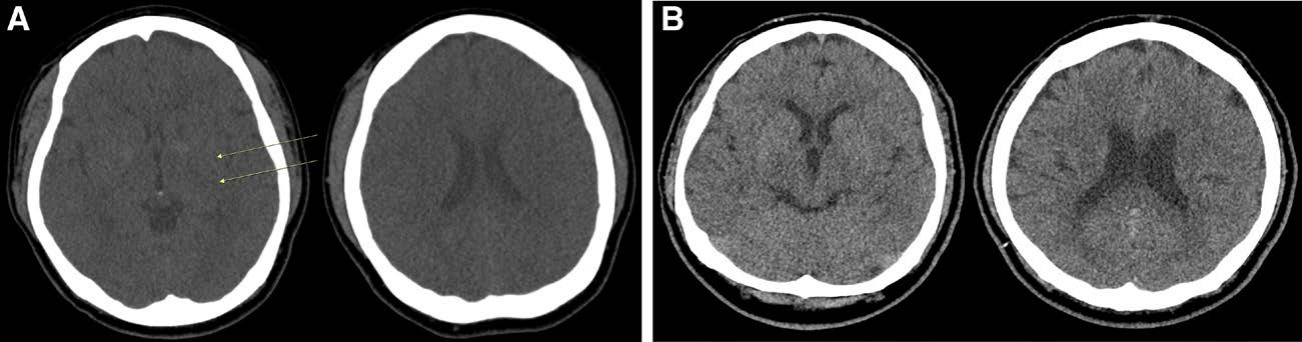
Examples of the Alberta Stroke Program Early Computed Tomography (ASPECT) score evaluation. (A) an example of a patient with a poor ASPECT score of 7, showing slightly decreased intensity in the left putamen, internal capsule, and insular cortex. (B) an example of a patient with a good ASPECT score of 10, showing no decreased intensity.
